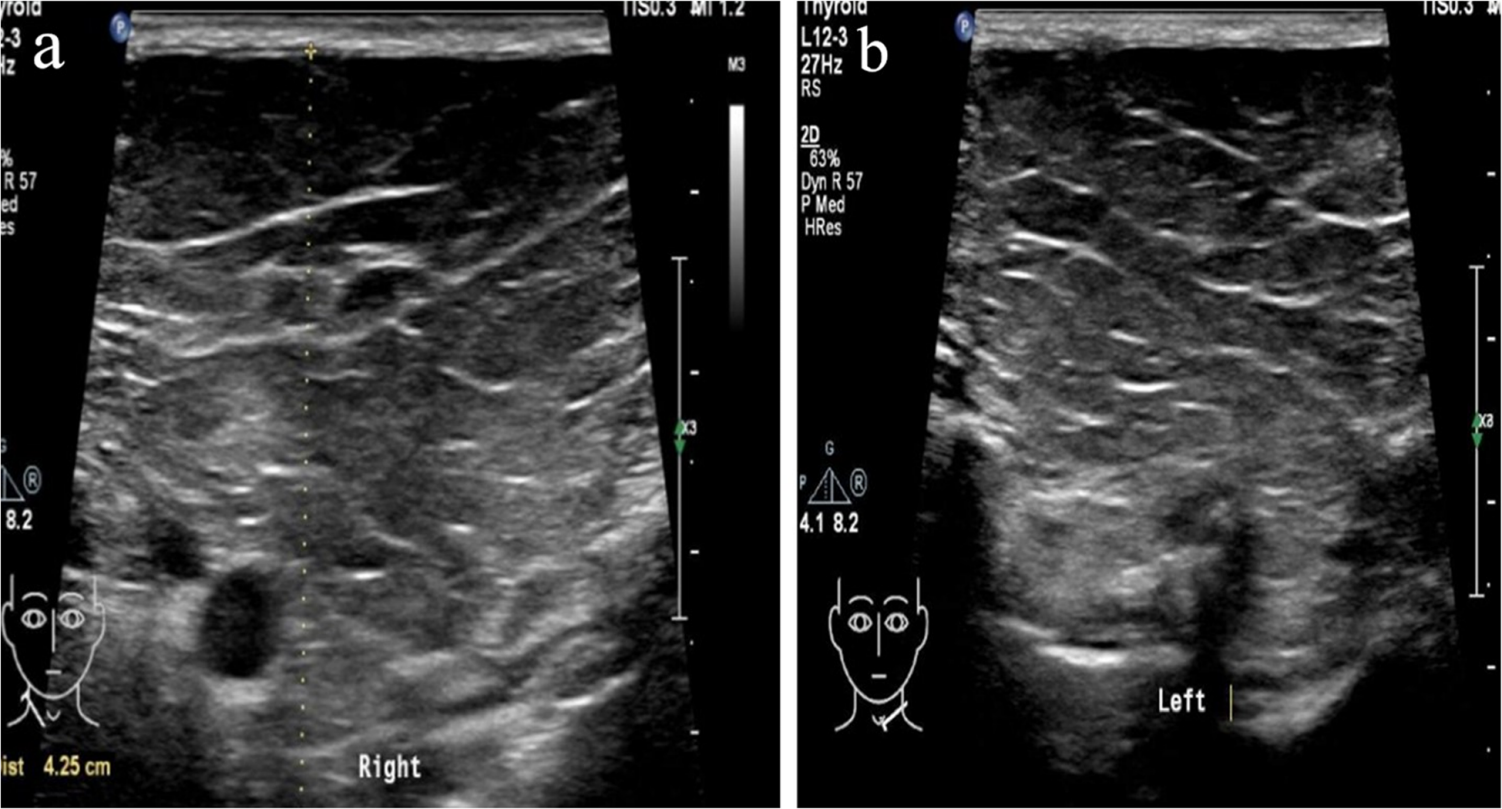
Ultrasound scan of the neck: diffuse hyperplasia of subcutaneous fat in neck and bilateral supraclavicular fossa, the thickest is 4.3 cm on the right and 4.5 cm on the left. a Right. b Left
